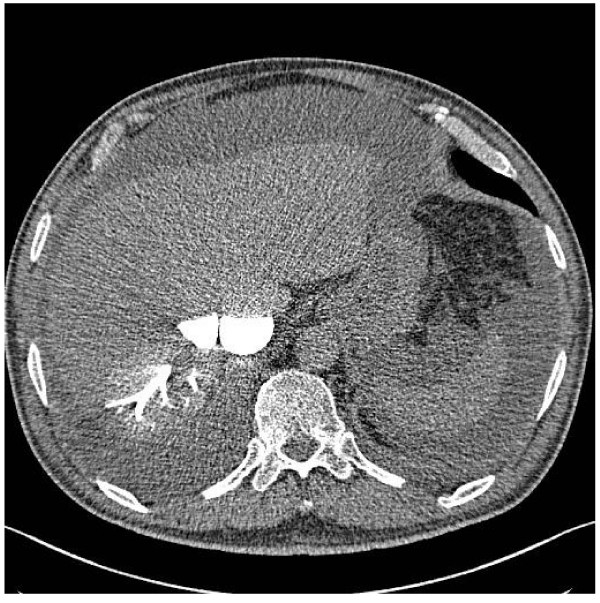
CT abdomen showing contrast in inferior vena cava and hepatic venous system.
